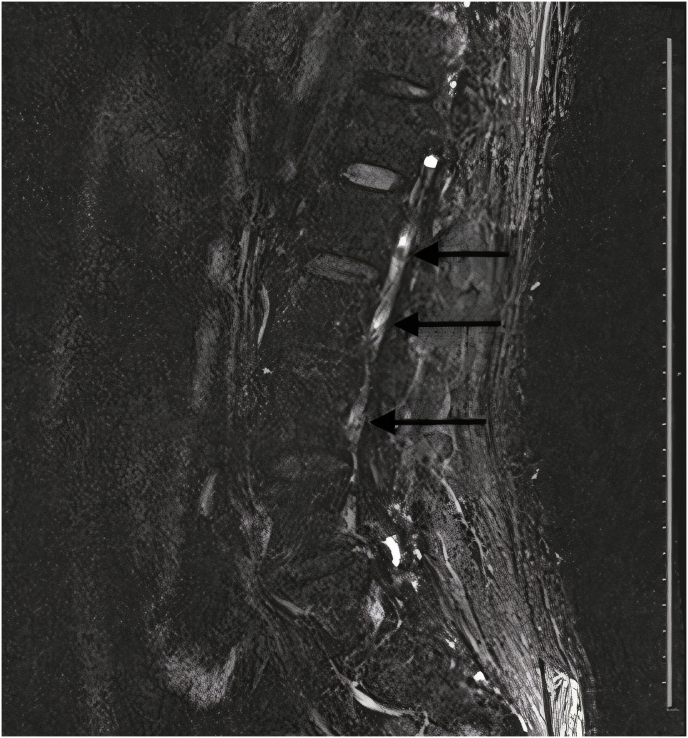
Magnetic resonance image of the spine with intravenous gadolinium showing bilateral contrast enhancement of the nerve roots in the lumbar region and the upper part of the cauda equina (as indicated by the arrows).
